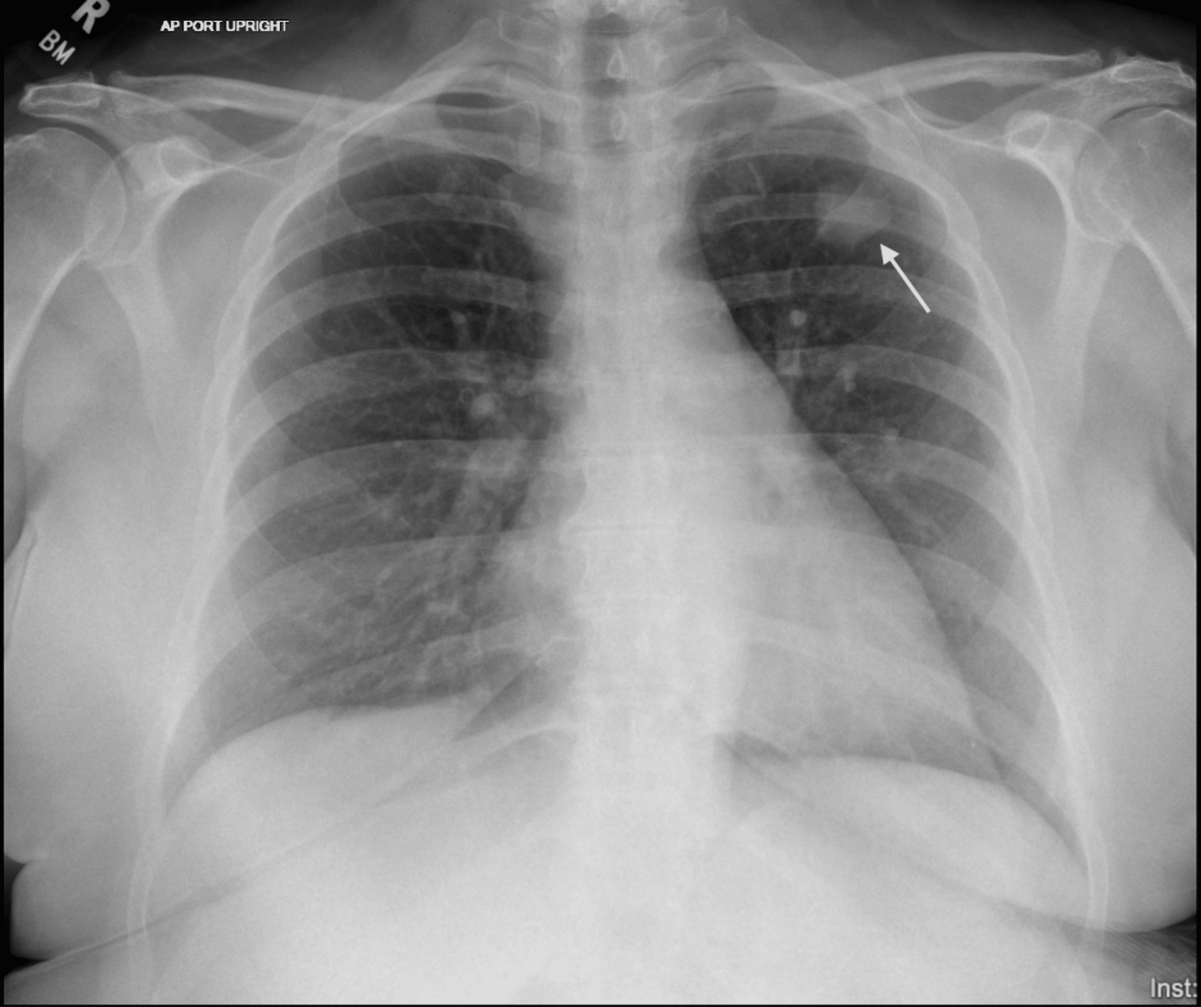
Chest X-ray showing a solitary lung nodule in the left lung (as highlighted by the arrow)
